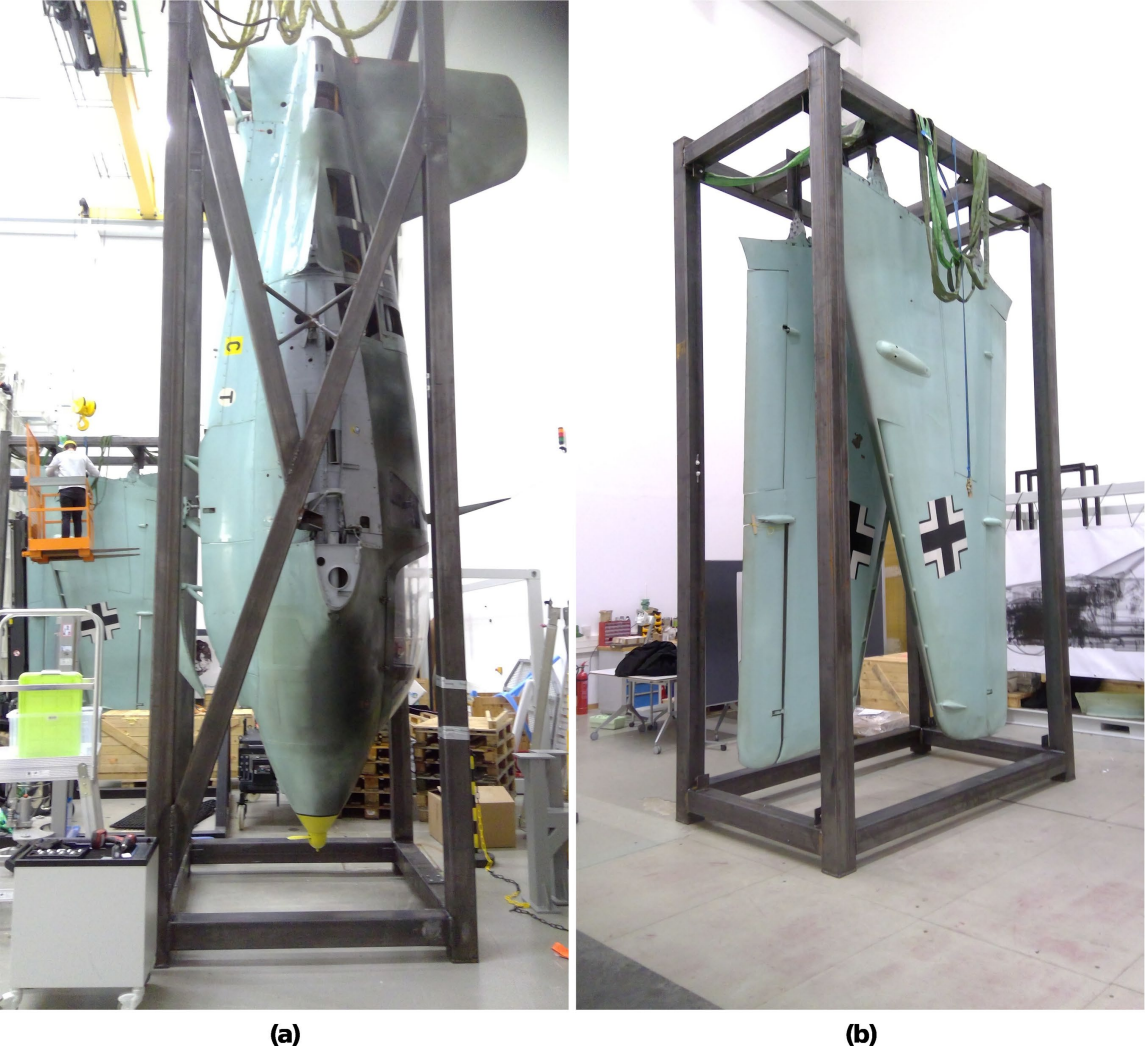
Fuselage (a) and wings (b) of the Me 163 airplane inside the mounting brackets for the CT scan.
